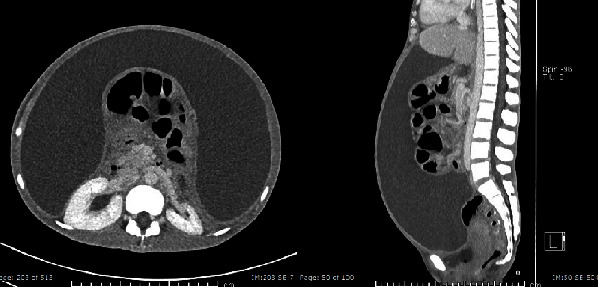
Computed tomography of the abdominopelvic region shows massive loculated ascites with internal thin septation, causing posterior displacement of the intraperitoneal organs.
